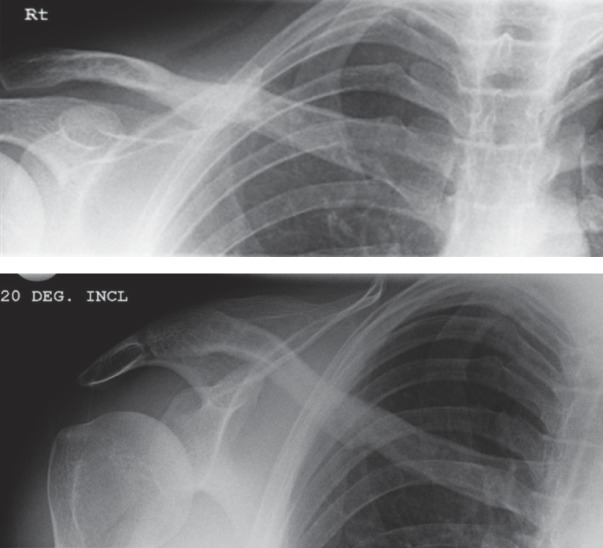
AP (top) and 20-degree (bottom) radiographs of the clavicle 6 months after surgery, showing union of the clavicle fracture.
